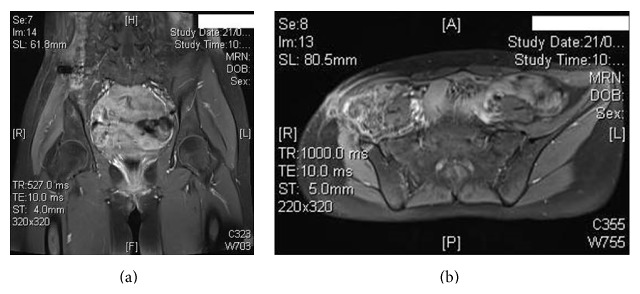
MRI images of the patient showing no evidence of tumor.
